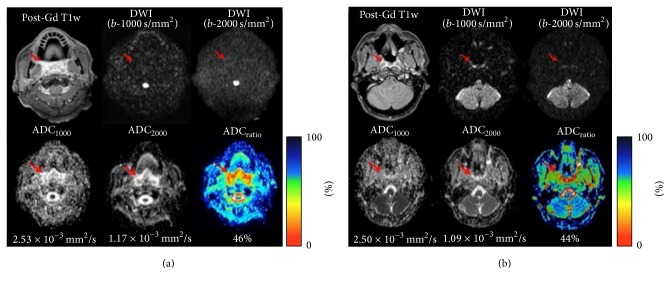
Representative MR images of posttreatment changes: (a) patient number 3 (M, 69 yo) after radiation therapy for an oropharynx cancer. Postcontrast T1-weighted sequence shows a diffuse and enhanced oropharyngeal thickening. Signal intensity is not increased on DW images at standard and high* b*-values. (b) Patient number 9 (M, 50 yo) after chemoradiotherapy for a nasopharyngeal cancer. Postcontrast T1-weighted sequence shows a right posterolateral thickening of nasopharynx. DW images at standard and high* b*-values depicted a mild high signal intensity (T2 shine-through effect). In both cases, posttreatment changes are characterized by high signal intensity on the corresponding ADC maps. Mean ADC_1000_, ADC_2000_, and ADC_ratio_ were 2.53 × 10^−3^ mm^2^/s, 1.17 × 10^−3^ mm^2^/s, and 46% (a) and 2.50 × 10^−3^ mm^2^/s, 1.09 × 10^−3^ mm^2^/s, and 44% (b).
